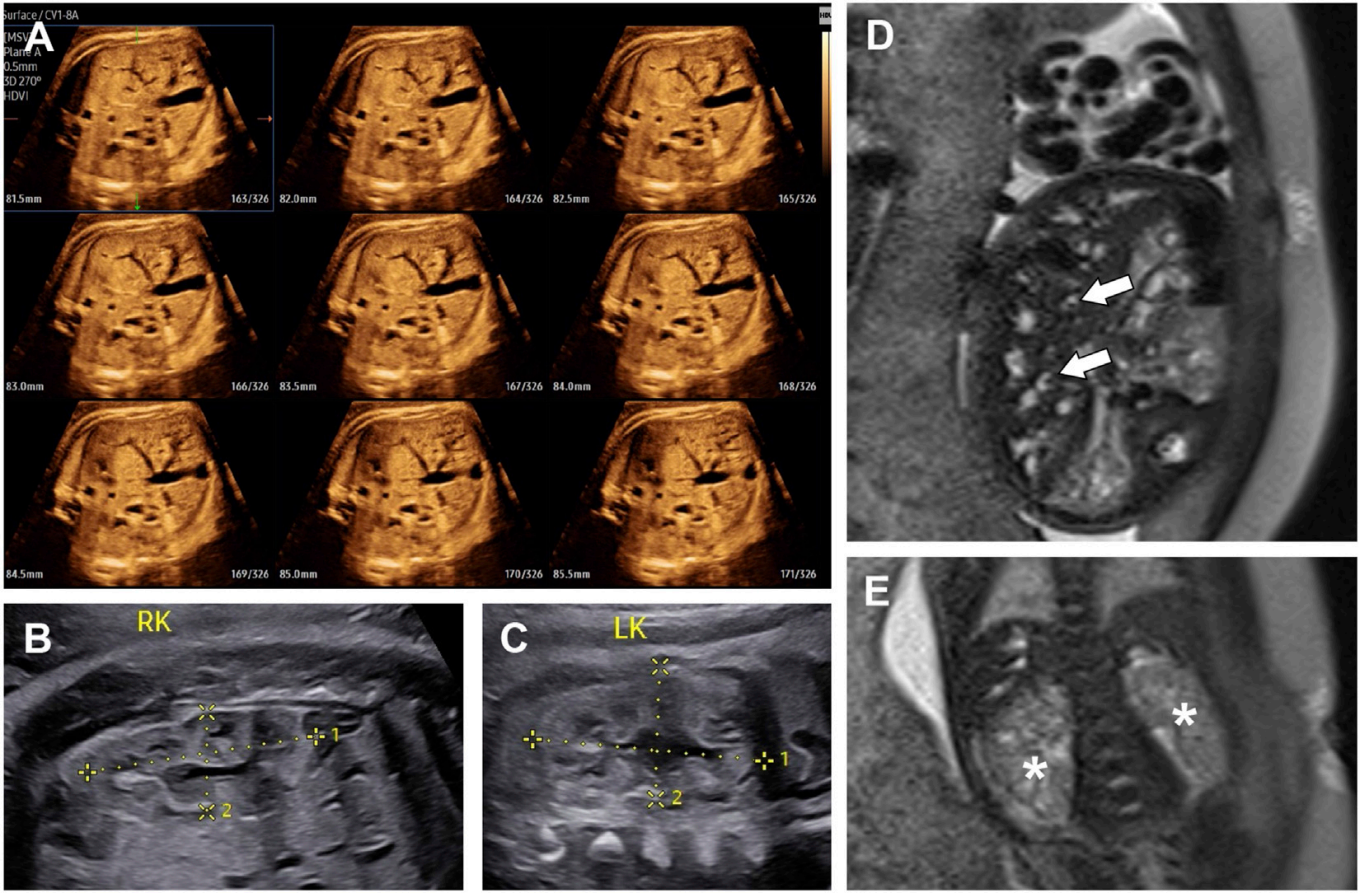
The ultrasound images (A–C) and T2-weighted MRI images (D,E) of fetal abdomen at 33+4 weeks of gestation. (A) Multi-Slice view of fetal liver, showing multiple small tubular and cystic anechoic structures. (B) Right kidney (4.06 × 1.75 cm). (C) left kidney (3.88 × 2.22 cm). Both kidneys showed no obvious enlargement and loss of corticomedullary differentiation. (D) The heterogeneous liver with multiple dilated bile ducts. The central dot sign was indicated by arrows. (E) Two slightly enlarged kidneys (asterisks), showing numerous tiny cysts and no corticomedullary differentiation.
